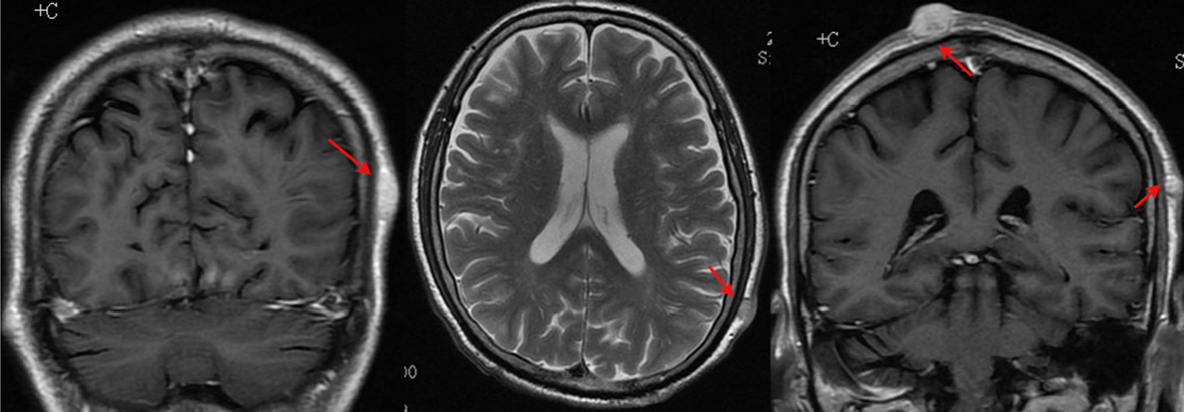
Head MRI showed multiple lesions in the soft tissue of the scalp.
